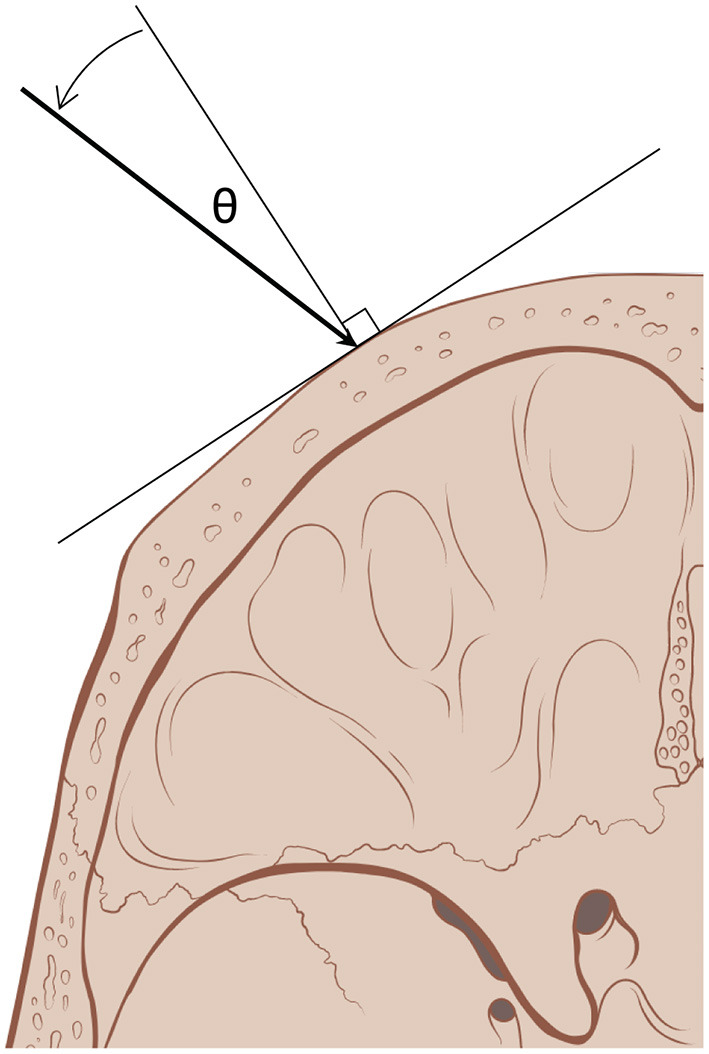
Incident angle (θ) measures the degree from normal, orthogonal, at which acoustic beams (arrow) emitted by ultrasound elements reach the outer table of the skull. With a smaller incident angle, a greater proportion of the beam's energy traverses the skull (skull art reused with permission from Patrick J. Lynch and C. Carl Jaffe, MD).
